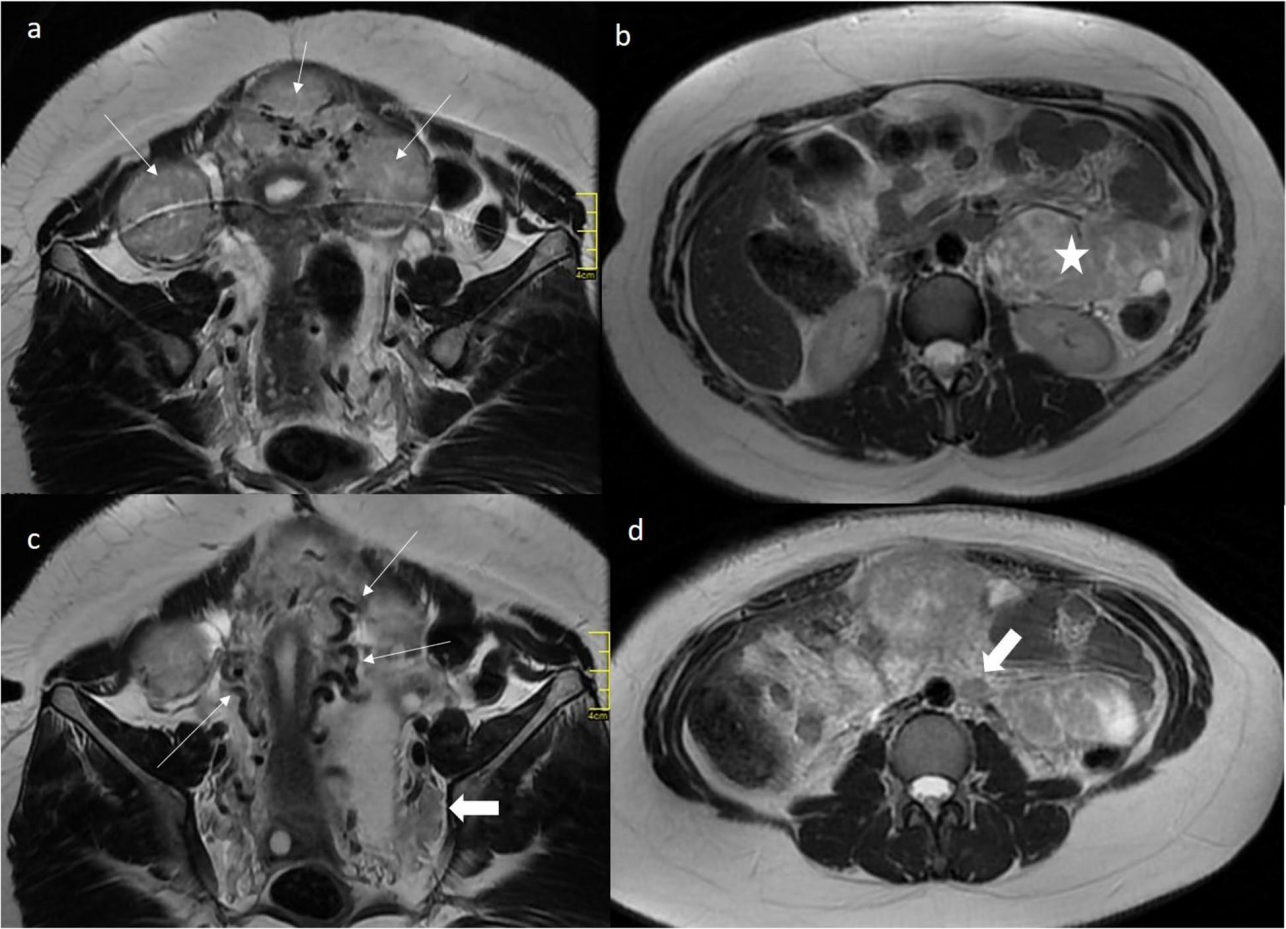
a Coronal T2-weighted MRI showing three well-circumscribed hypointense intramural and subserosal leiomyomas (thin arrows), b Axial T2-weighted MRI showing interloop and left kidney polypoid mass (indicated by ★) and (c) lymphadenopathies in left iliac chain (thick arrows). The bridging vessels sign confirms the uterine origin of the mass (thin arrows), (d) lymphadenopathie in lateroaortic chain (thick arrow)
